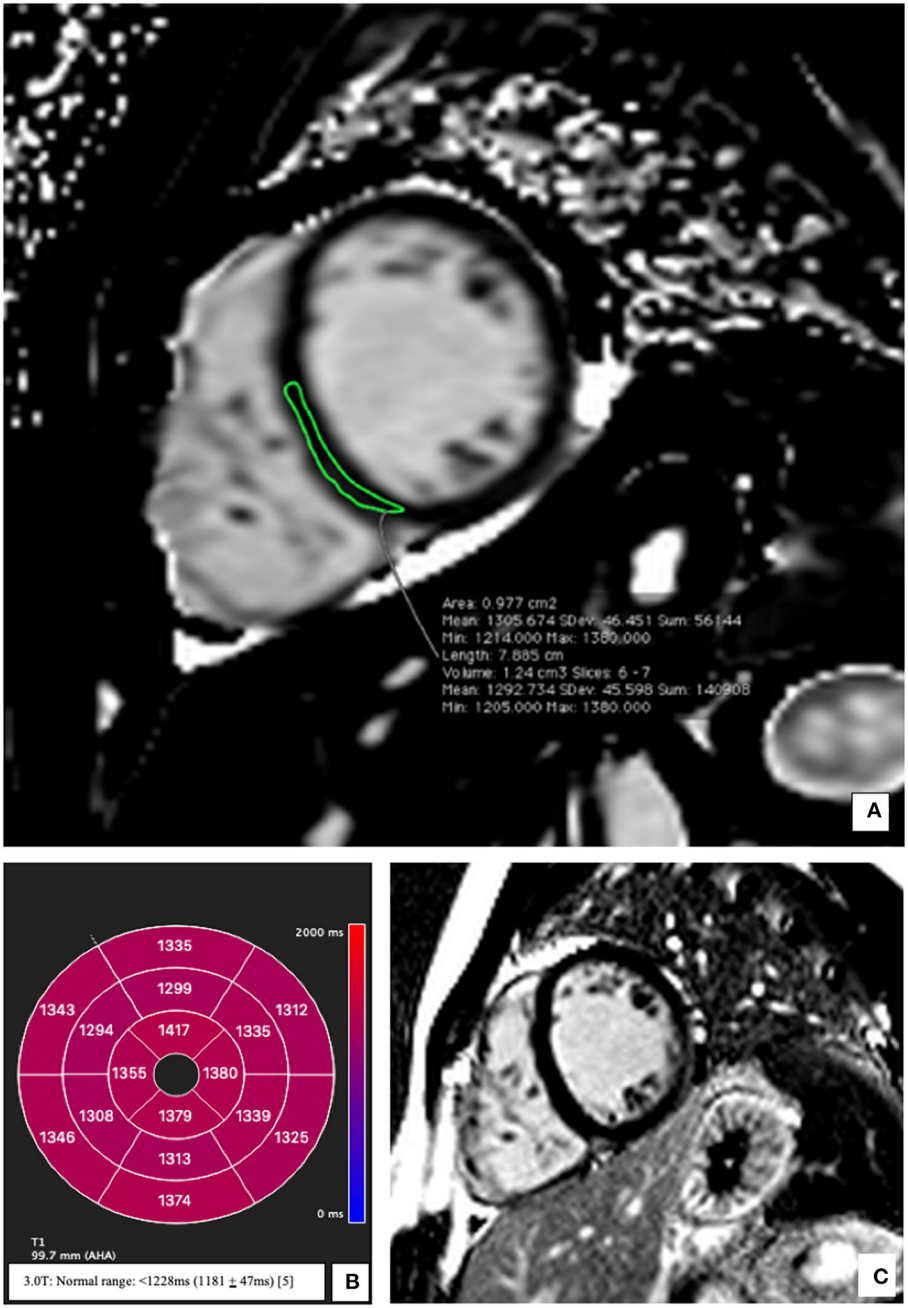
Representative native T1 mapping image and the corresponding late gadolinium enhancement (LGE) image at presentation. (A) Midventricular short axis image of T1 map (SAX) showing elevation of the T1 times; an example from the inferoseptal segment (demarcated green area: 1,305 ms). (B) Bullseye plot showing the native T1-times. Segmentation was performed according to the AHA 16-segment model. Native T1 times were increased in all segments irrespective of the presence/absence of LGE. (C) Corresponding mid-ventricular SAX LGE image without evidence of LGE. Normal T1 Mapping values for a 3 Tesla MRI <1,228 ms (1,181 ± 47 ms) (5).
